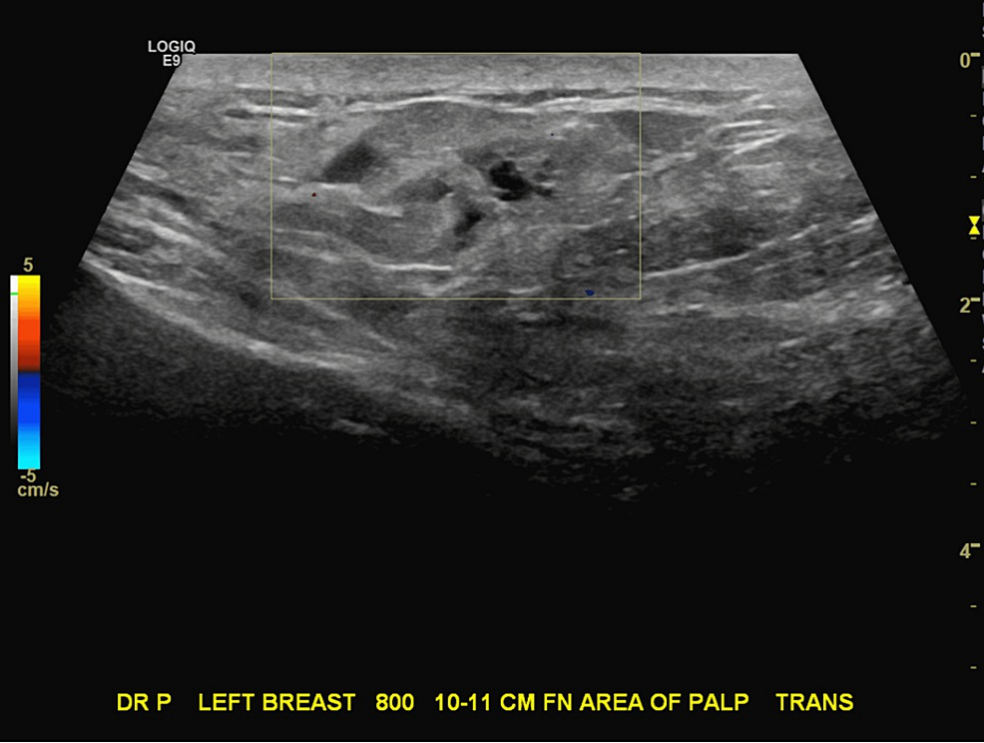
Ultrasound of Left Breast Mass with Color Doppler (Transverse View) This focused Doppler ultrasound image (transverse view) of the palpable left breast mass, taken approximately 10-11 cm from the nipple at the eight o'clock position, reveals a prominent fat lobule measuring 6 cm x 2 cm with an area of hypoechoic change along the edge of the lobule that is avascular and has the sonographic appearance of probable fat necrosis.
